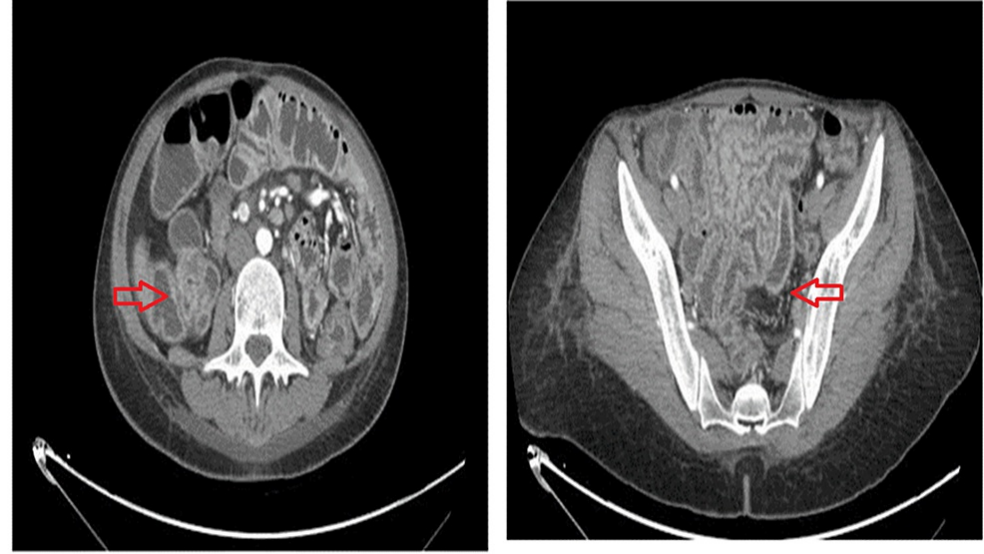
CT showing the increased enhancement of gastric, jejunal, and colonic mucosa, which demonstrated an inflammatory process, particularly in the jejunum and to lesser extent in the terminal ileum. CT: computed tomography
